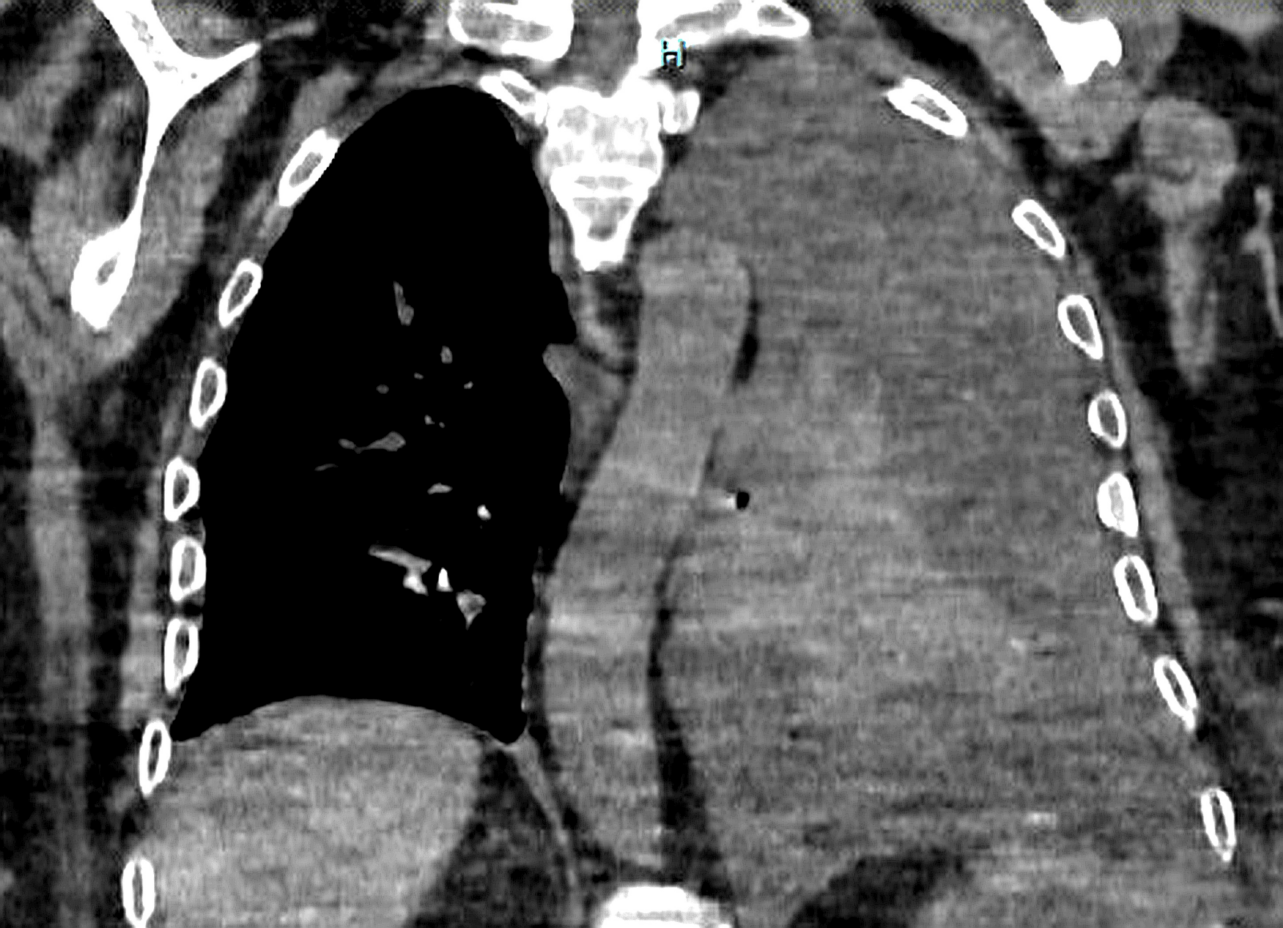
A chest CT scan revealed a large left pyothorax compressing the lung
